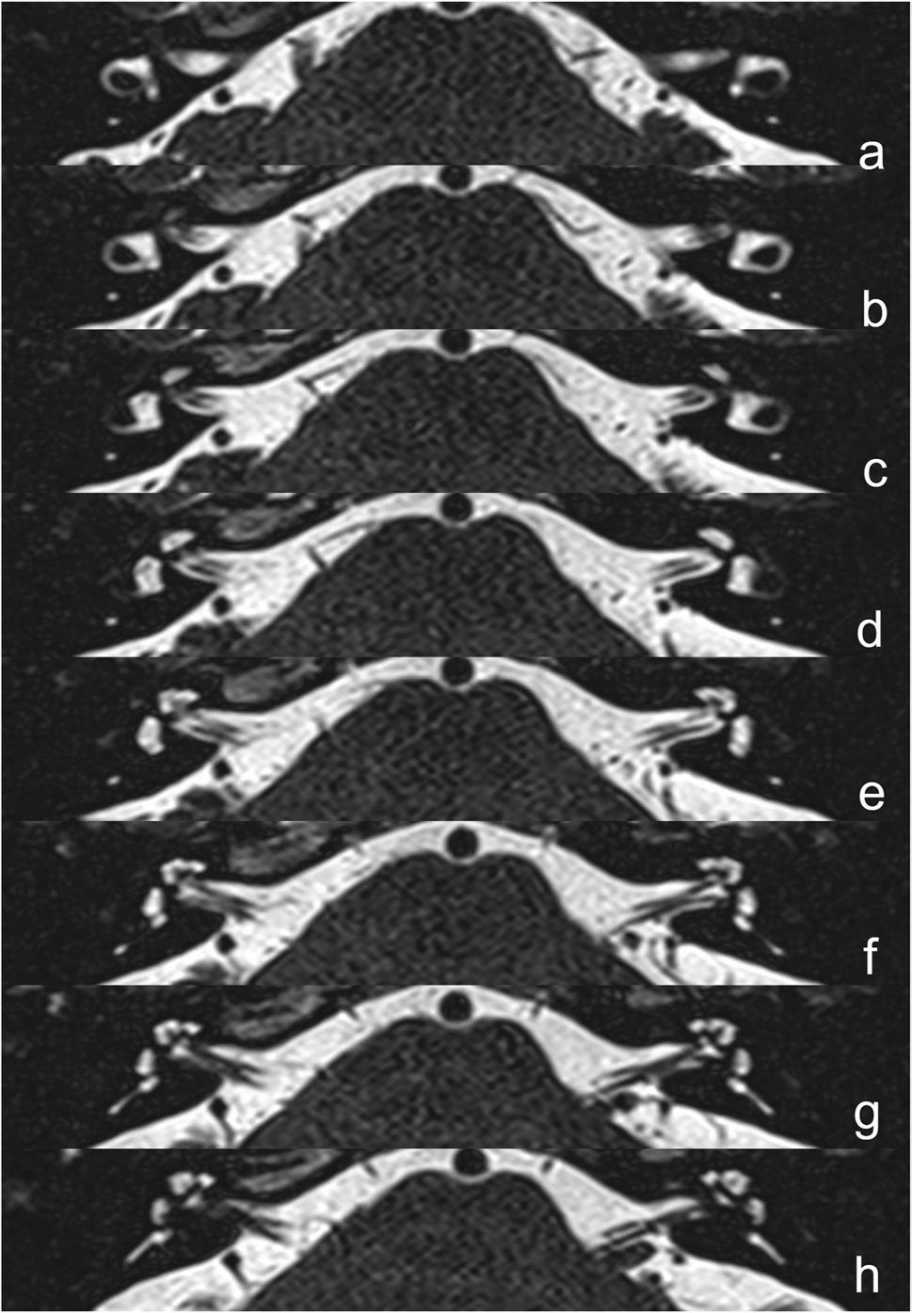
3D-SPACE MRI images of a 53-year-old male with left-sided unilateral MD. (a, b, c, d, e, f, g, h) Axial, high-resolution, T2-weighted MRI scan showing non-visualization of the vestibular aqueduct on both sides
